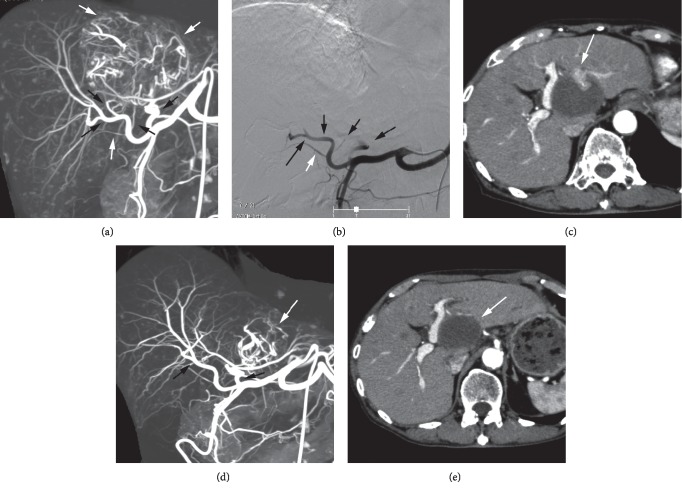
RAIB-TACE for a large nodule in the liver hilum. Immediately before the first session of RAIB-TACE, MIP image of trans-arterial MDCT angiography via the celiac artery well depicted hepatic arterial branches and the large nodule (large white arrow, A) in the liver hilum. Multiple tiny high density spots were arterioportal shunts. RAIB-TACE was performed for right hepatic (large black arrow, A), medial segmental (small black arrow, A), caudate segmental (small black arrowhead, A), and left hepatic arteries (large black arrowhead, A). Post-TACE DSA showed occlusion of all hepatic arterial branches (black arrows and arrowheads, B) and patent cystic artery (white arrowhead, B). CT 3 months after RAIB-TACE depicted a remaining viable part (white arrow, C), and PR was achieved. The second session of RAIB-TACE was performed 5 months after the first one. MIP image of trans-arterial MDCT angiography via the celiac artery showed patent hepatic arterial branches (black arrow, black arrowhead, D) and tumor vessels in a remaining viable part of the large nodule (white arrow, D). RAIB-TACE could be successfully done via the left hepatic artery (black arrowhead, D). CR of the large nodule (white arrow, E) was achieved after the second session of RAIB-TACE.
